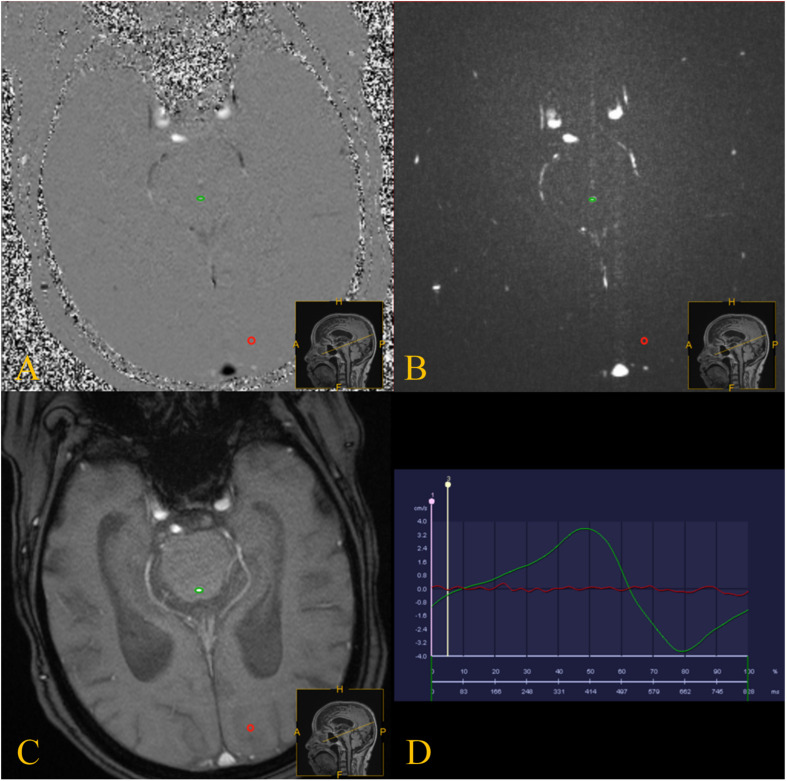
PC-MRI was performed with the slice orientation perpendicular to the aqueduct. (A–C) PC-MRI with a manually drawn region of interest (green circle) defining the aqueduct, and the reference region of interest is shown by the red circles. (D) the red line represents the flow of the reference region of interest, and the green line represents the CSF flow though the aqueduct.
